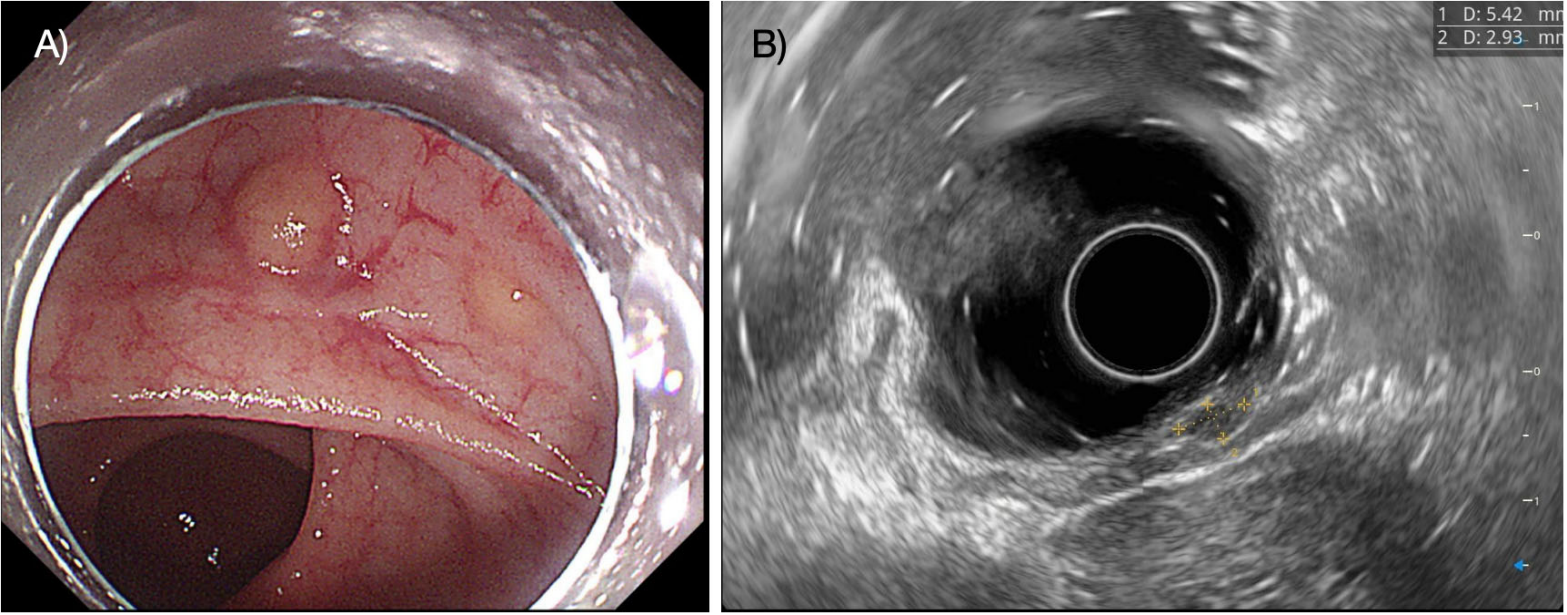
The figure shows two flat, yellowish lesions in the rectum (A), and endoscopic ultrasound reveals that the lesions are located in the submucosal layer (B).
